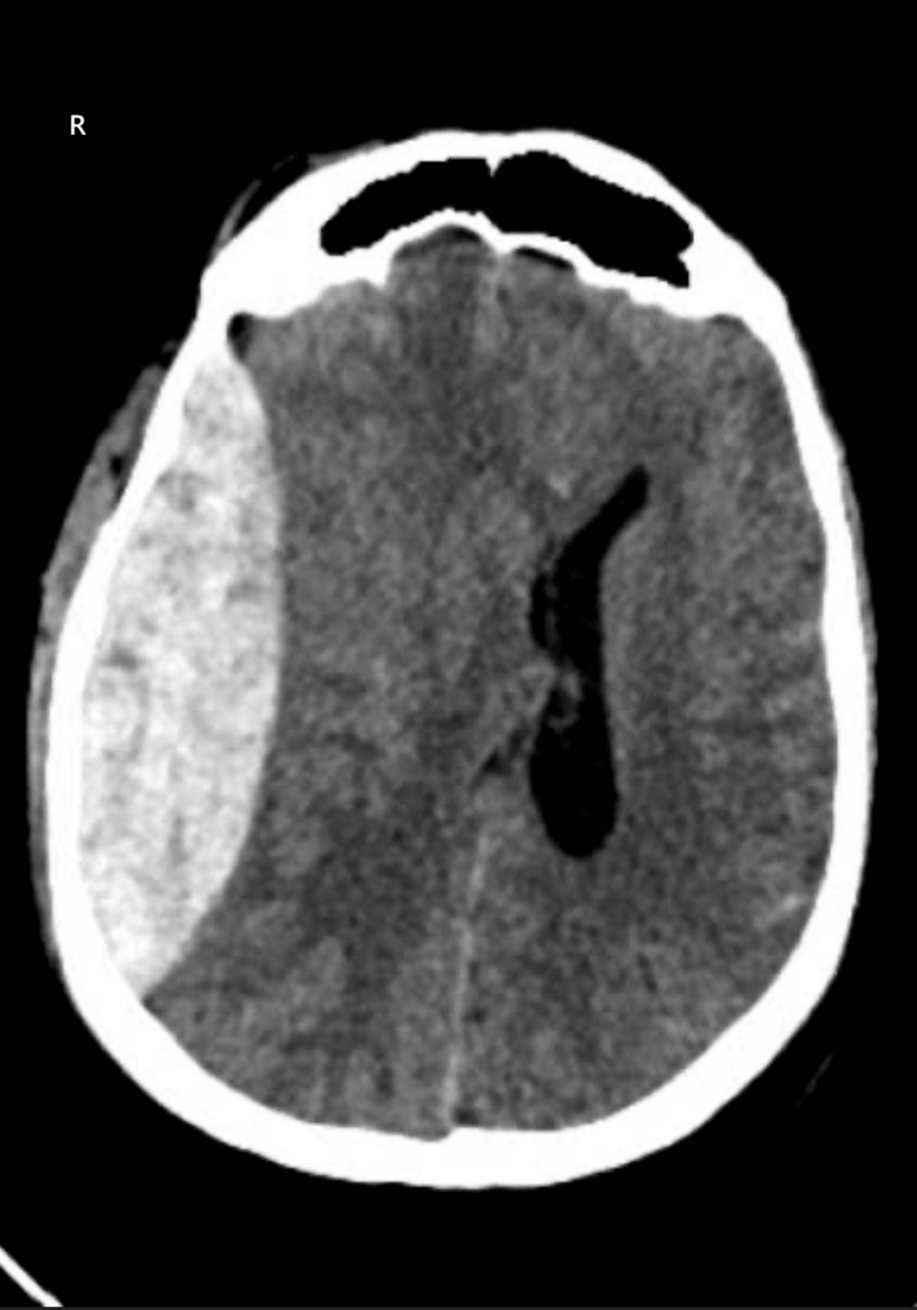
Non-contrast axial CT of the head showing right frontoparietal epidural hematoma Non-contrast axial CT of the head showing a large biconvex (lentiform), hyperdense collection along the right frontoparietal convexity in the extra-axial location, causing mass effect with midline shift, consistent with an acute epidural hematoma in the right frontoparietal region.
